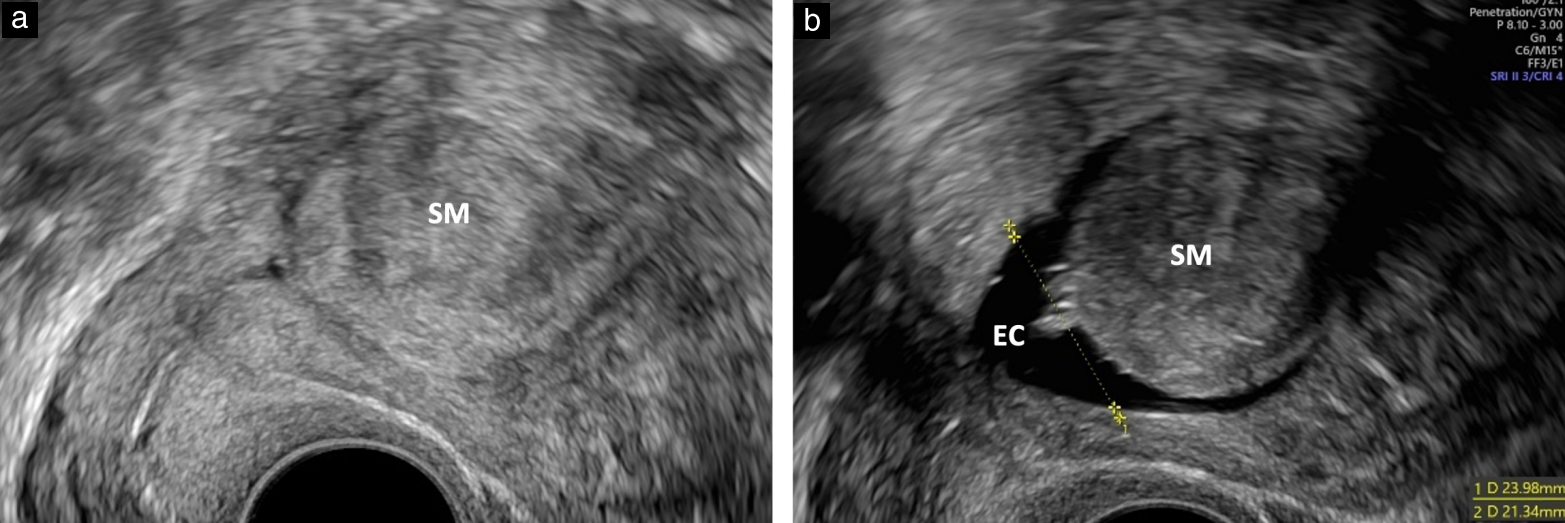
Visualization of endometrium in presence of submucous fibroid (SM) on transvaginal ultrasound (a) and on saline contrast sonohysterography (multimodal approach) (b). Calipers indicate measurement technique. EC, endometrial cavity.
